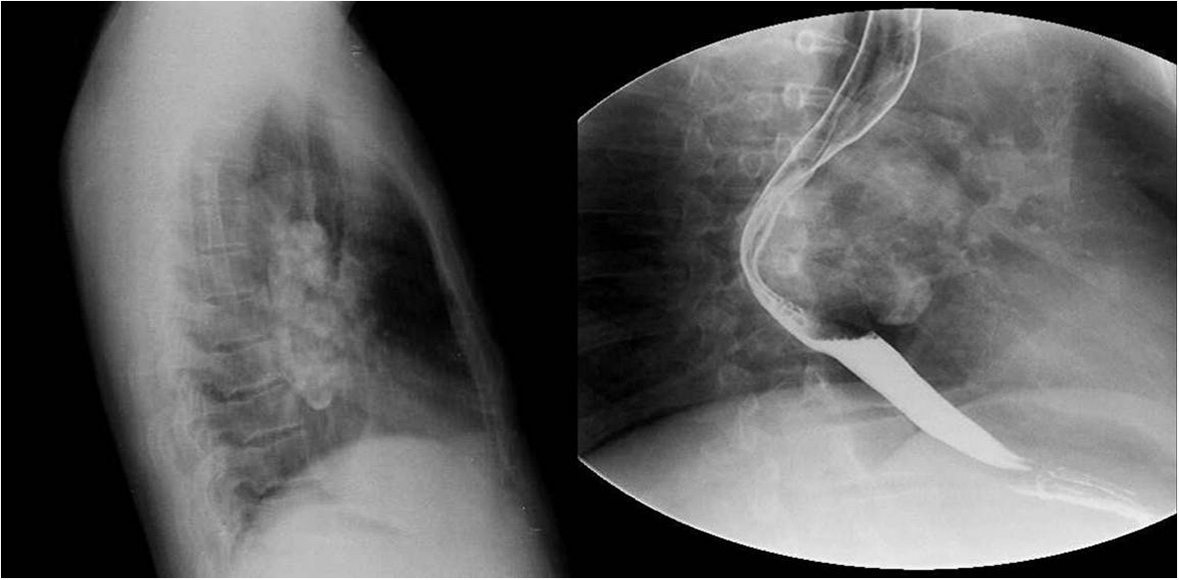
A chest lateral radiograph shows a large mass with lots of calcification in the mediastinum; esophagus barium opacification shows that the middle and inferior segment of the esophagus was compressed by the mass.
